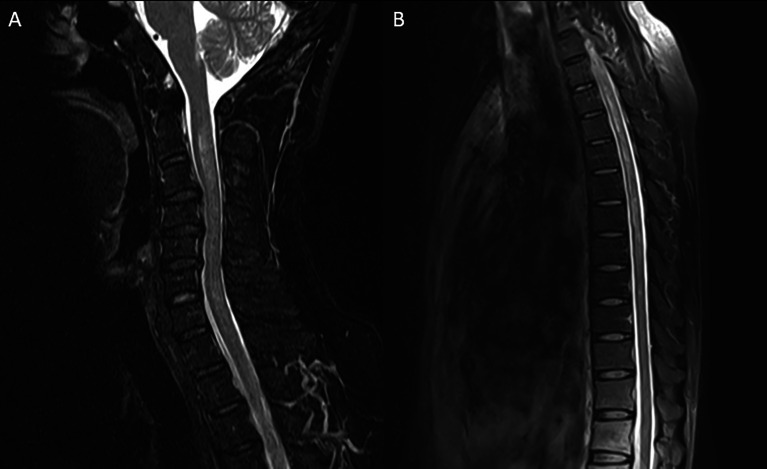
Magnetic resonance imaging of the cervical (A) and thoracic (B) spinal cord. Sagittal T2-weighted imaging showed longitudinally extensive hyperintense lesion extending from C2 to T8.
